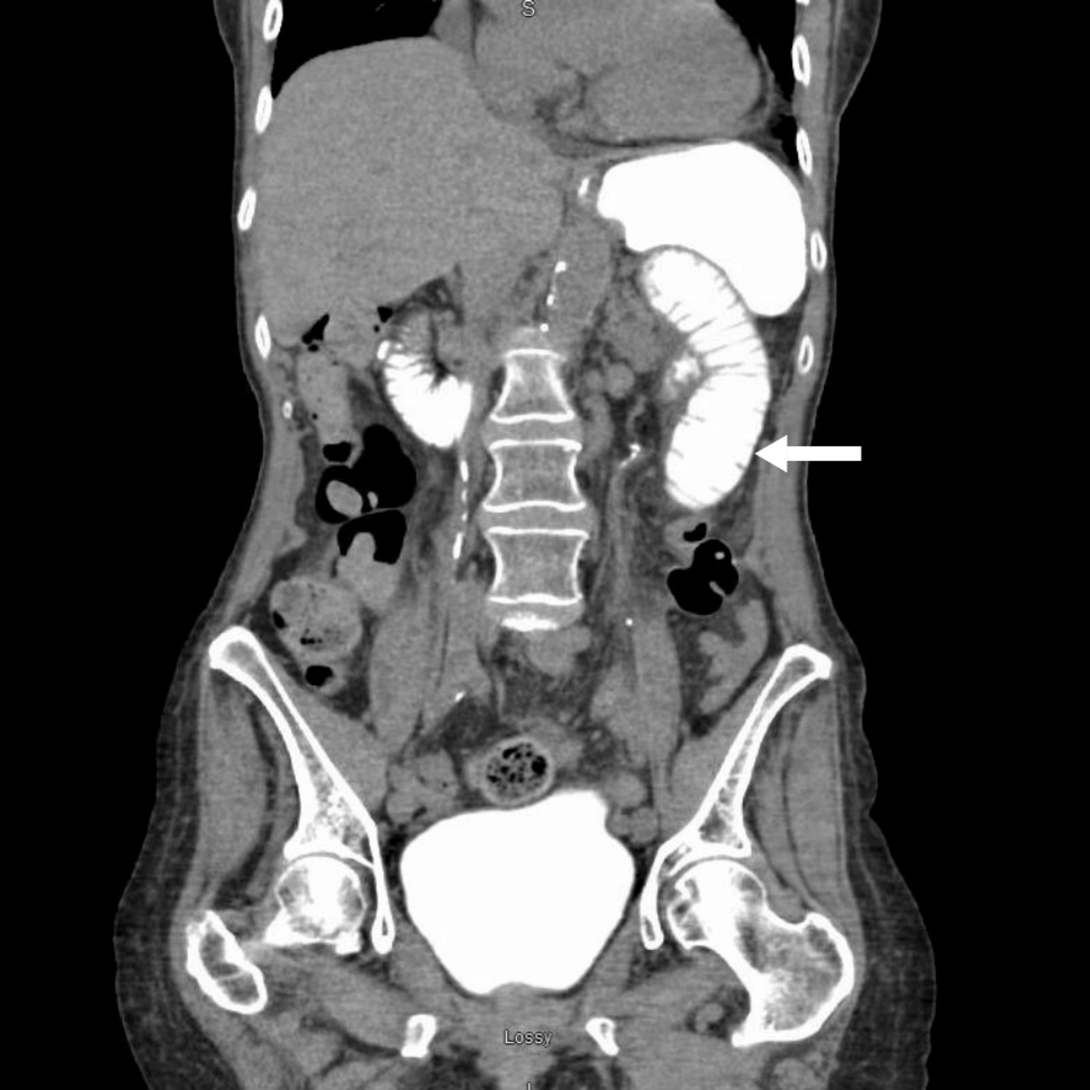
Coronal view of a contrast-enhanced computed tomography (CT) scan of the abdomen and pelvis (with intravenous and oral contrast). Demonstrating findings concerning small bowel obstruction versus ileus, with contrast reaching the proximal small bowel (white arrow).
